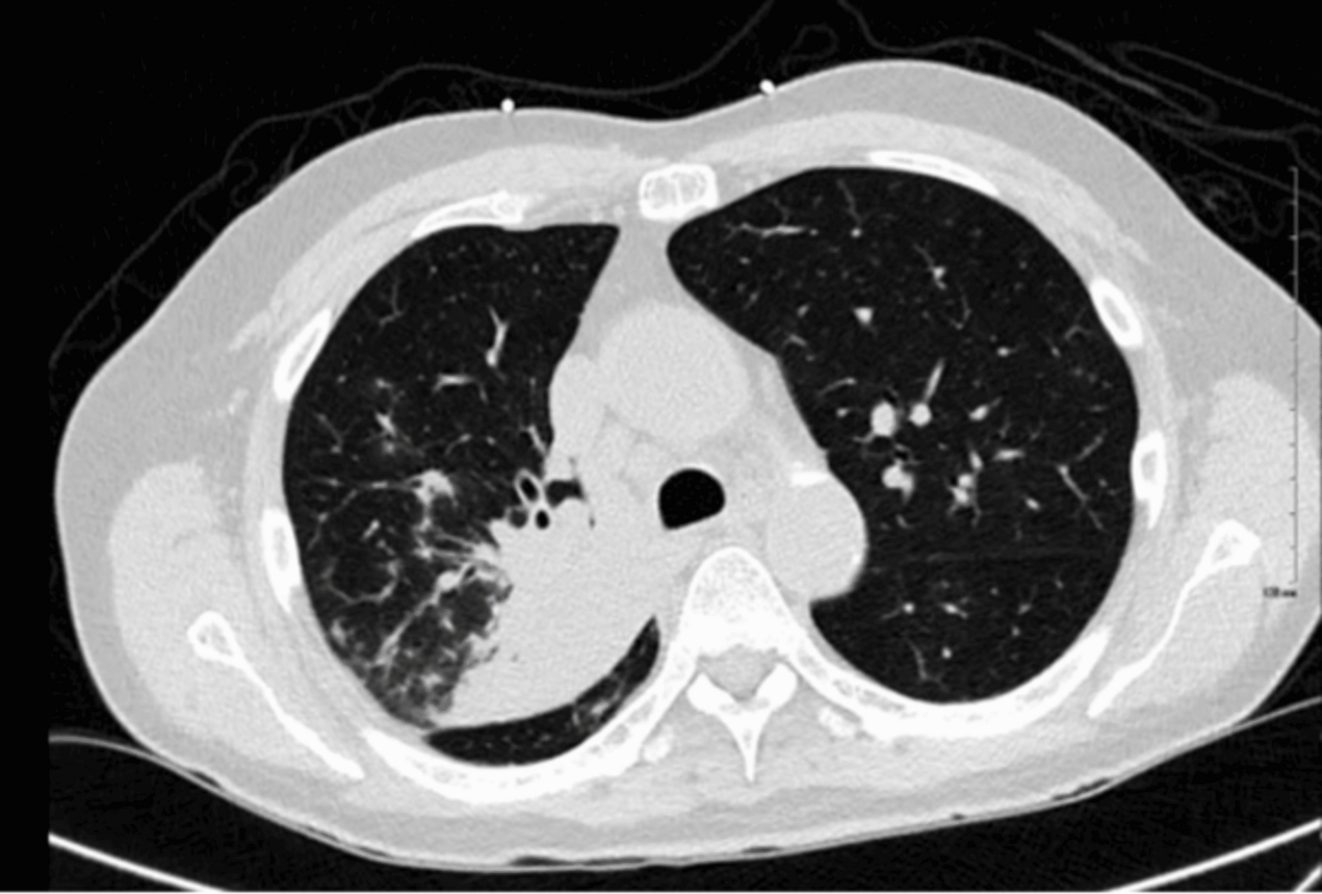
CT scan of the chest without contrast demonstrated a confluent right upper lobe infiltrate with posterior segment consolidation.
